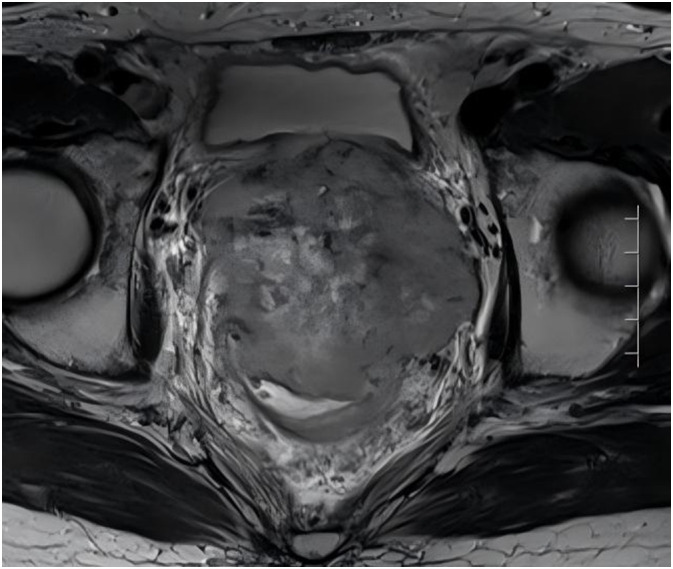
Prostate MRI (3.0T). The prostate is enlarged with an ill-defined lesion.
